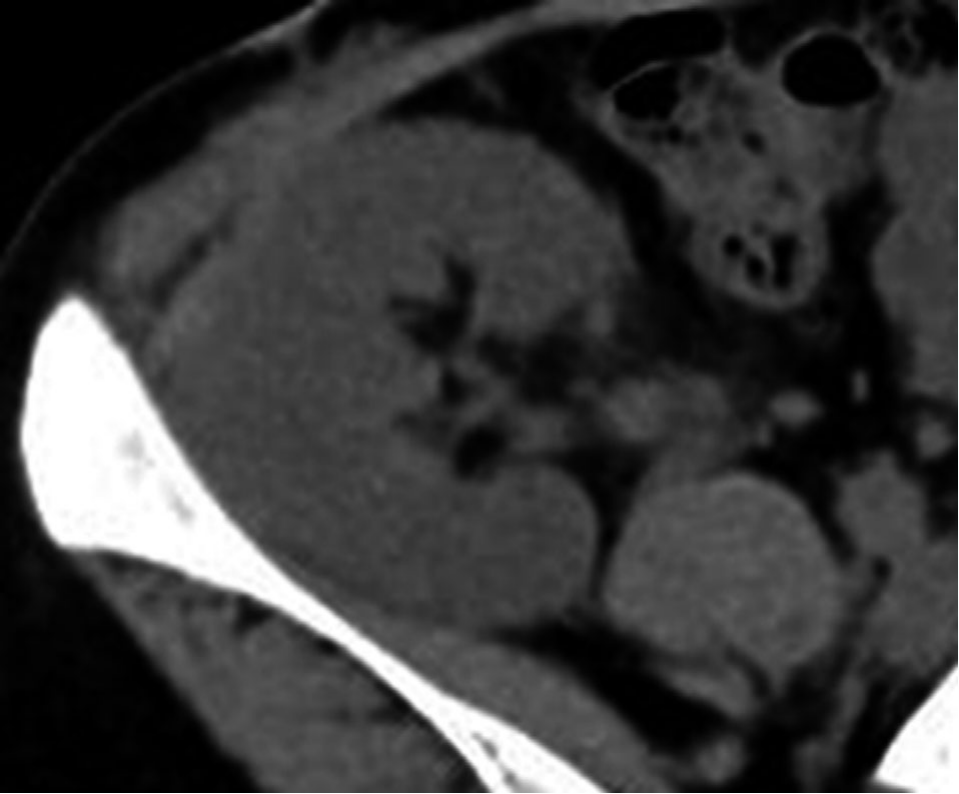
Representative image of allograft 12 months after LDKT. Abdominal CT showing that the graft had no calcification 1 year after LDKT. Urine calcium levels gradually decreased after LDKT, and graft calcification did not occur.
